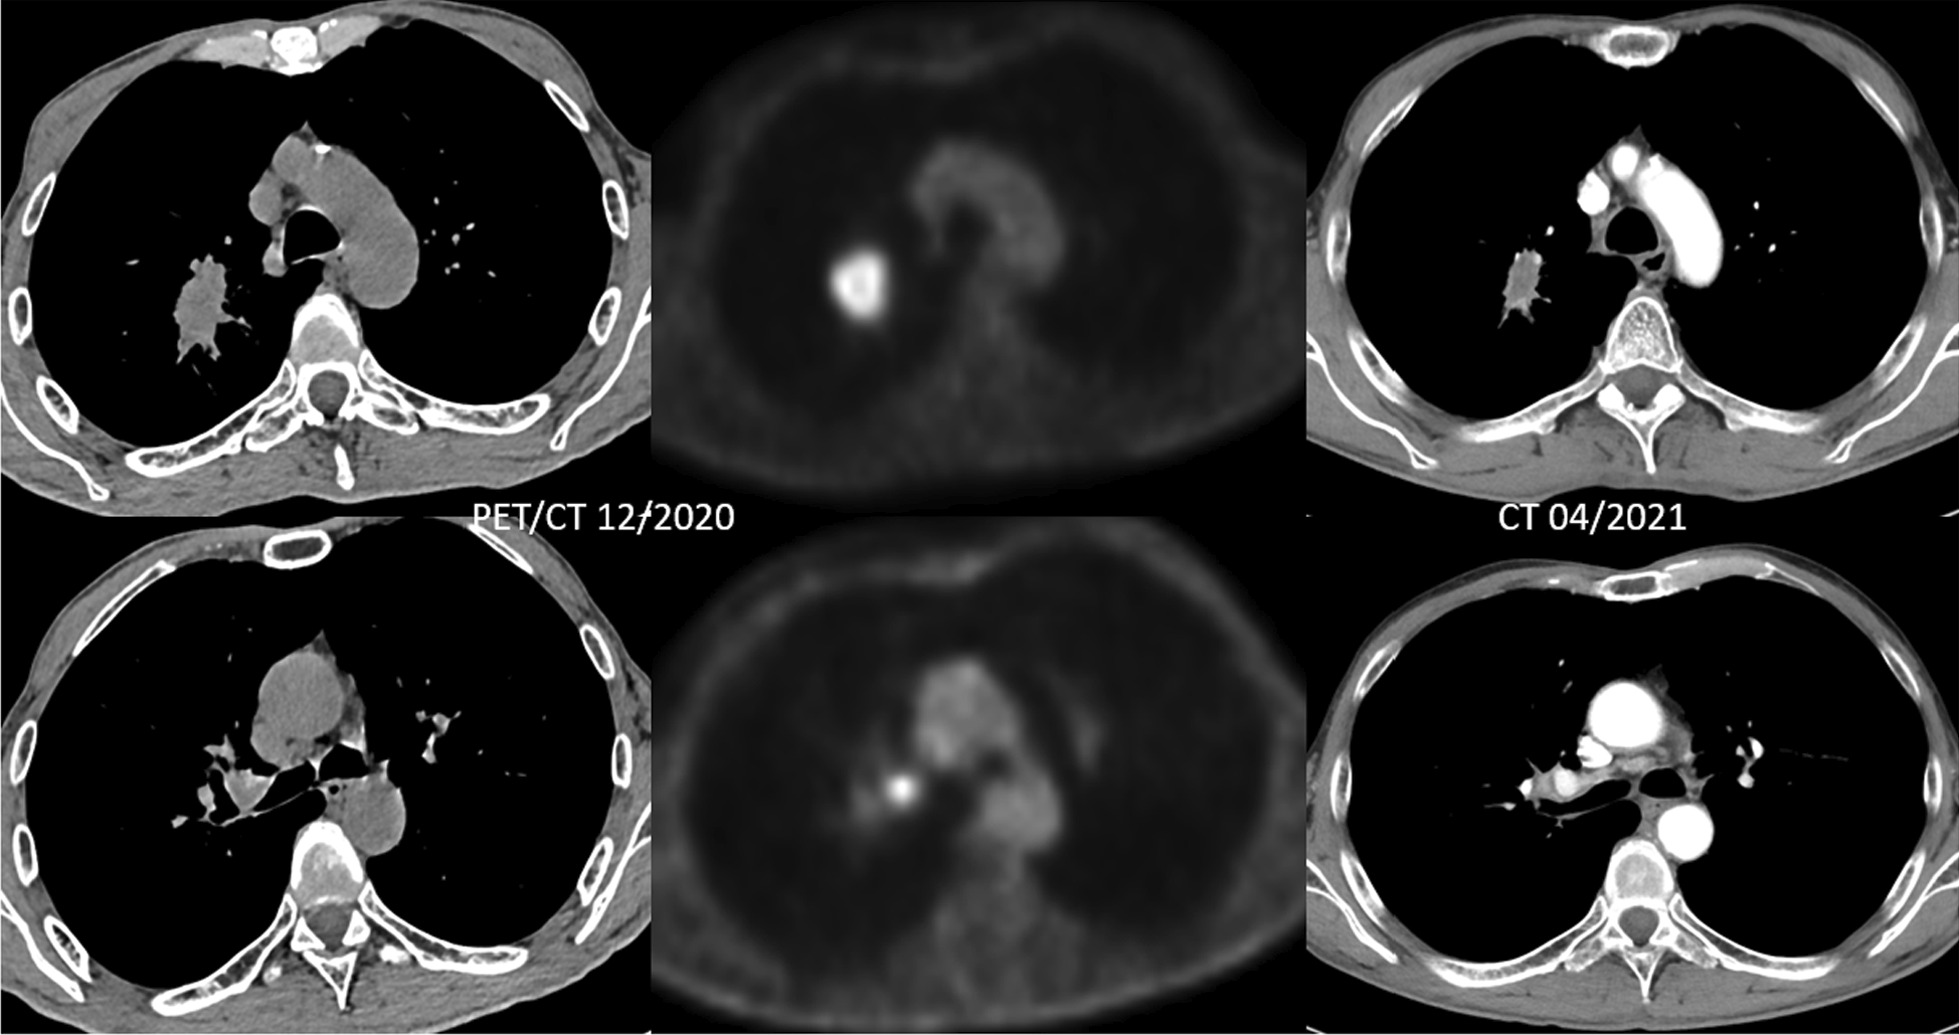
PET/CT at initial diagnosis 12/2020; note enhanced FDG-uptake in the primary tumour (SUVmax 7) and LN pos. 11R (SUVmax 4) and Follow-up CT 3 months post-MRgRT in 04/2021 demonstrating a partial remission
